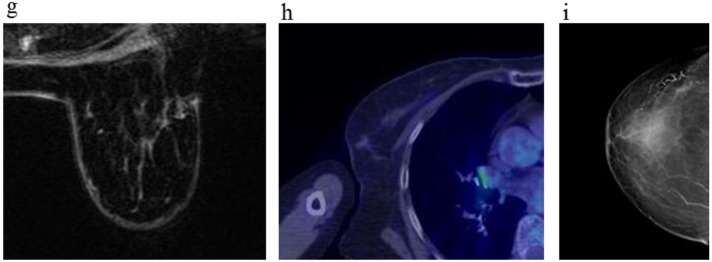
A 81-year-old female (case 7 in Table 1, Table 2 and Table 3) with right breast cancer (cT2N1M0). CE breast MRI, PET-CT and mammography revealed a breast tumor before KORTUC II therapy, the region of tumor was 25 mm in large diameter (a–c); At the initial examination after the completion of KORTUC II treatment, the tumor was undetectable on PET-CT and mammography (e,f); On the other hand, the residual tumor was still recognized on breast CE MRI ((d): arrows). However, complete response was eventually achieved and no recurrence was identified on final examinations of all of these three diagnostic modalities (g–i).
